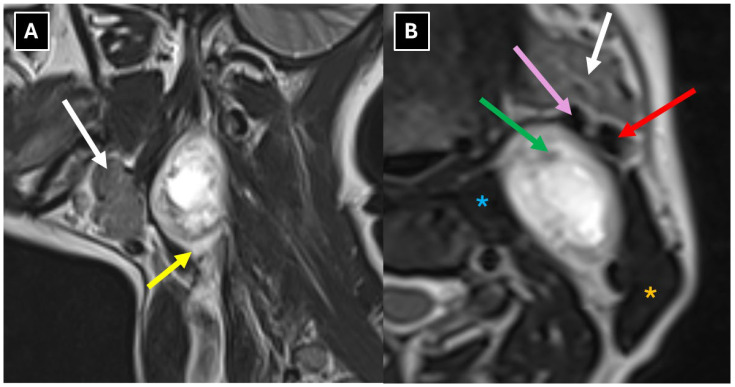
MRI scan of the neck. (A) Sagittal T2-weighted sequence shows a hyperintense, thin peripheral fat rim representing the “split-fat sign”. (B) Axial T2-weighted TSE Dixon (in) shows a round hypointense structure with a peripheral high signal—“fascicular sign”. Yellow arrow—“split-fat sign”; green arrow—“fascicular sign”; white arrows—parotid gland; red arrow—internal carotid artery; pink arrow—external carotid artery; orange asterisk—sternocleidomastoid muscle; blue asterisk—longus.
